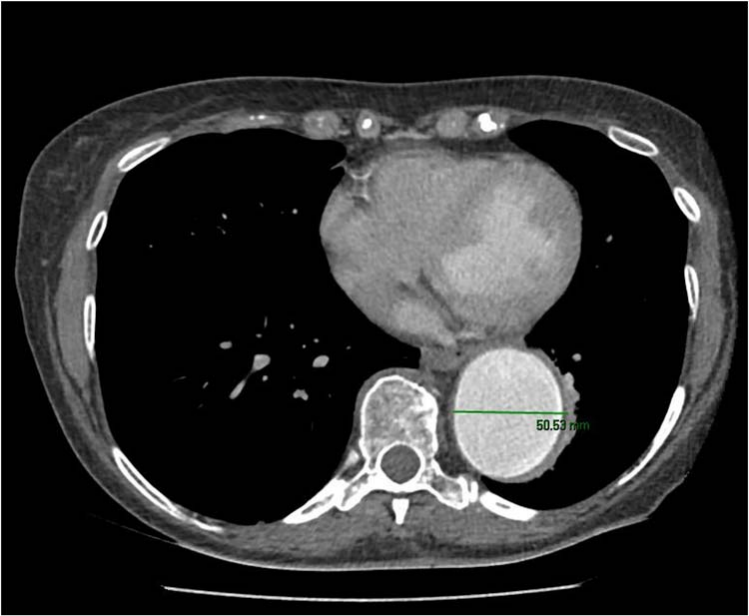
CT scan showing diameter of descending aorta measuring 50 mm.
